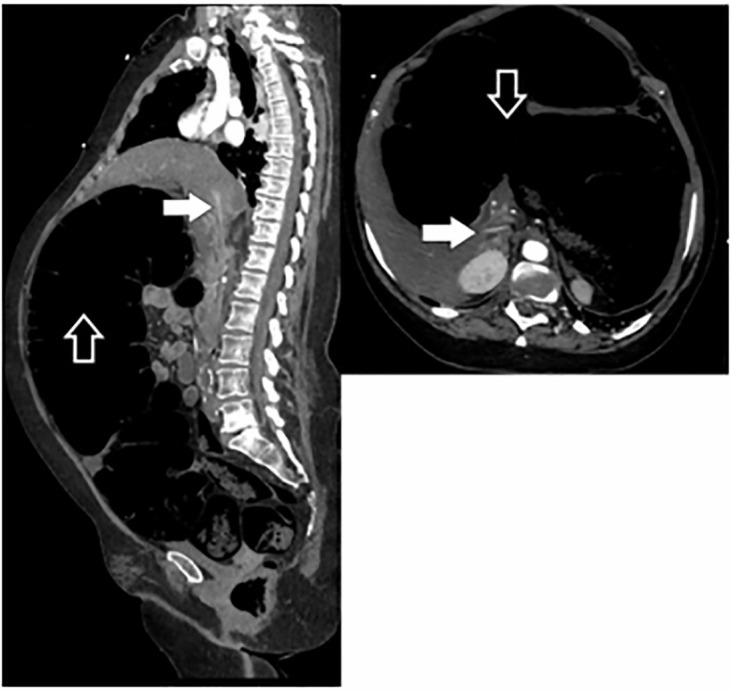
Sagittal (left) and axial (right) windows of the computed tomography
